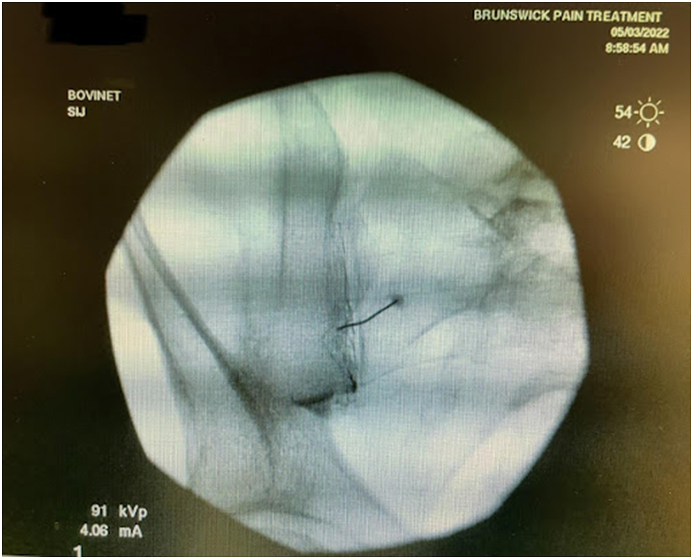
Contralateral oblique fluoroscopic image of needle trajectory. This figure depicts the needle from a diagnostic block and how the trajectory should be the same as the scout line extending across the joint on sagittal images of MRI or CT.
